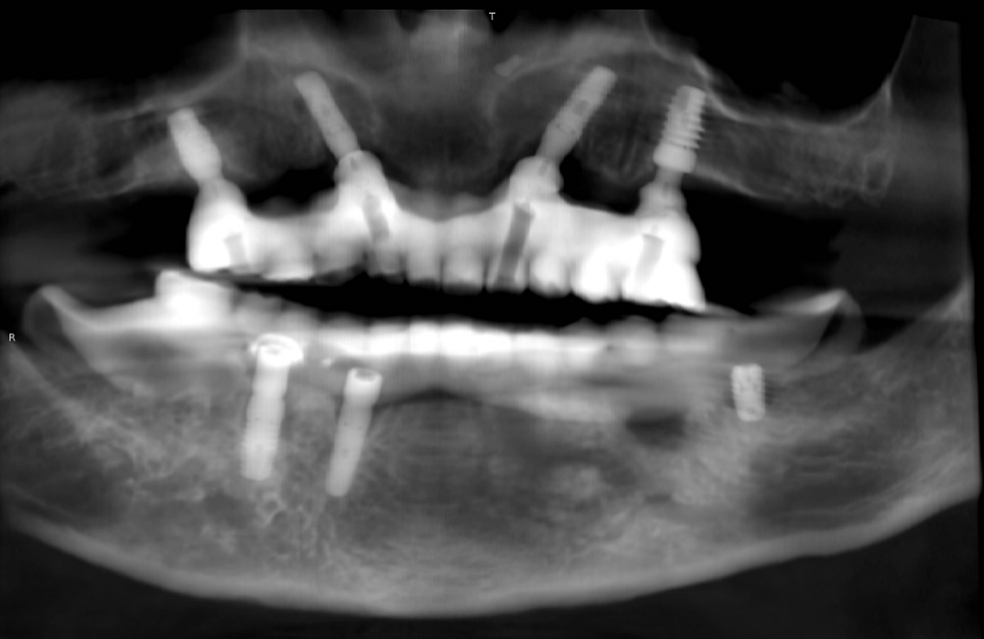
Panoramic reconstruction of the CBCT image at delivery for evaluation of fit of OnX implant-supported prosthesis. CBCT, cone beam computed tomography.
